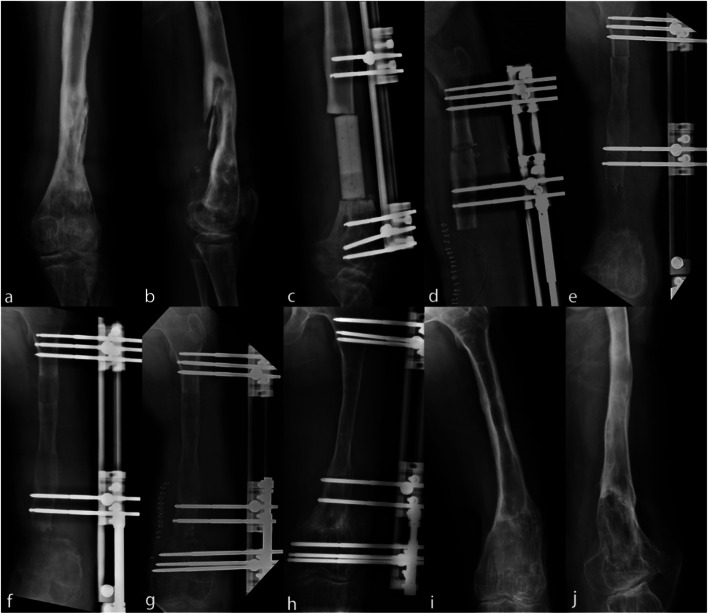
A 20-year-old male with post-traumatic osteomyelitis of the left femur was treated with single-level bone transport using external rail fixator. a, b Osteomyelitis of the left femur caused by post-traumatic infection presented by X-ray films. c, d, e Resection of infected bone with 7.8 cm defect, the antibiotic-impregnated cement spacer was filled and removed until infection was controlled, then treated by single-level bone transport. f Radiological films of 6 weeks in the distraction stage. g, h Bone transport completed with good regenerate consolidation, and docking union was achieved at four postoperative months. i, j External frame was removed with excellent bone result represented by view of X-ray at six postoperative months
